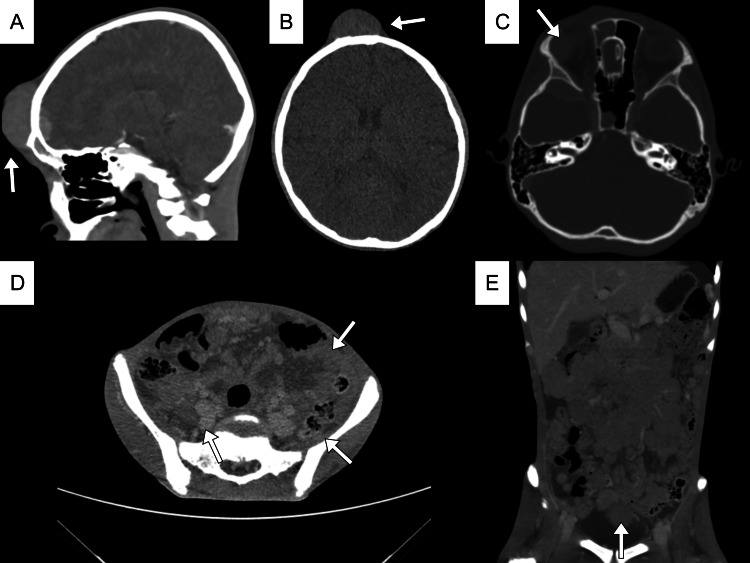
Brain and abdominal CT scan. (A-C) Brain CT scan shows multiple masses at the frontal bone, also found at the right intraorbital side of the eye. (D-E) Abdominal CT scan showed conglomerating masses at the paraaortic and mesentery areas extending to the pelvic region.
